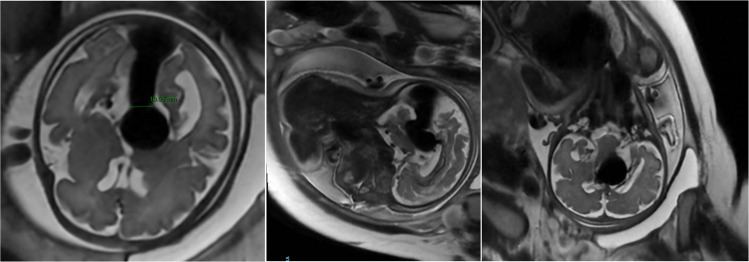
Fetal MRI of the clinical case demonstrating a vein of Galen malformation (VOGM) (Images from the present case)
